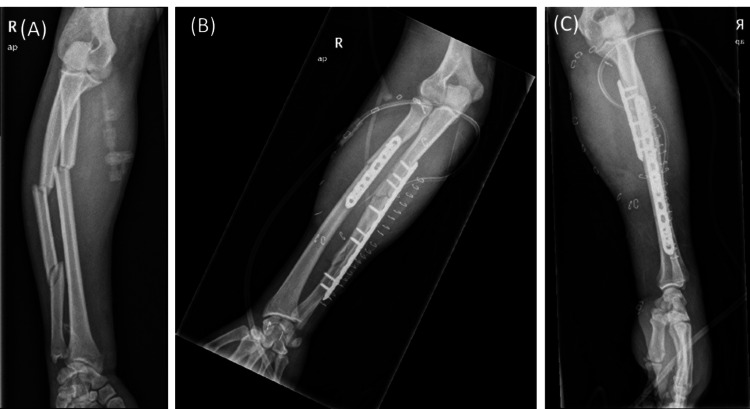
(A) Preoperative X-ray of a right forearm fracture, (B, C) Postoperative X-rays
